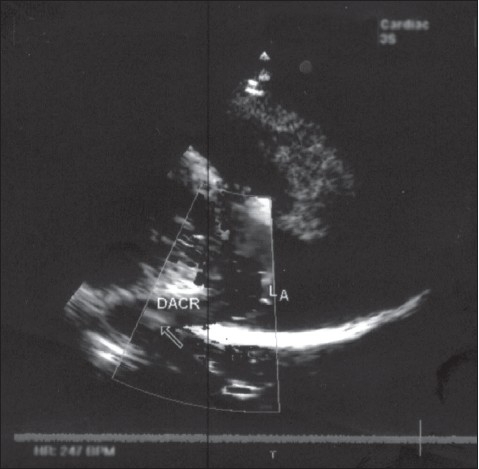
Trans-esophageal echocardiography showing the patent Dacron graft draining into the left atrium.
